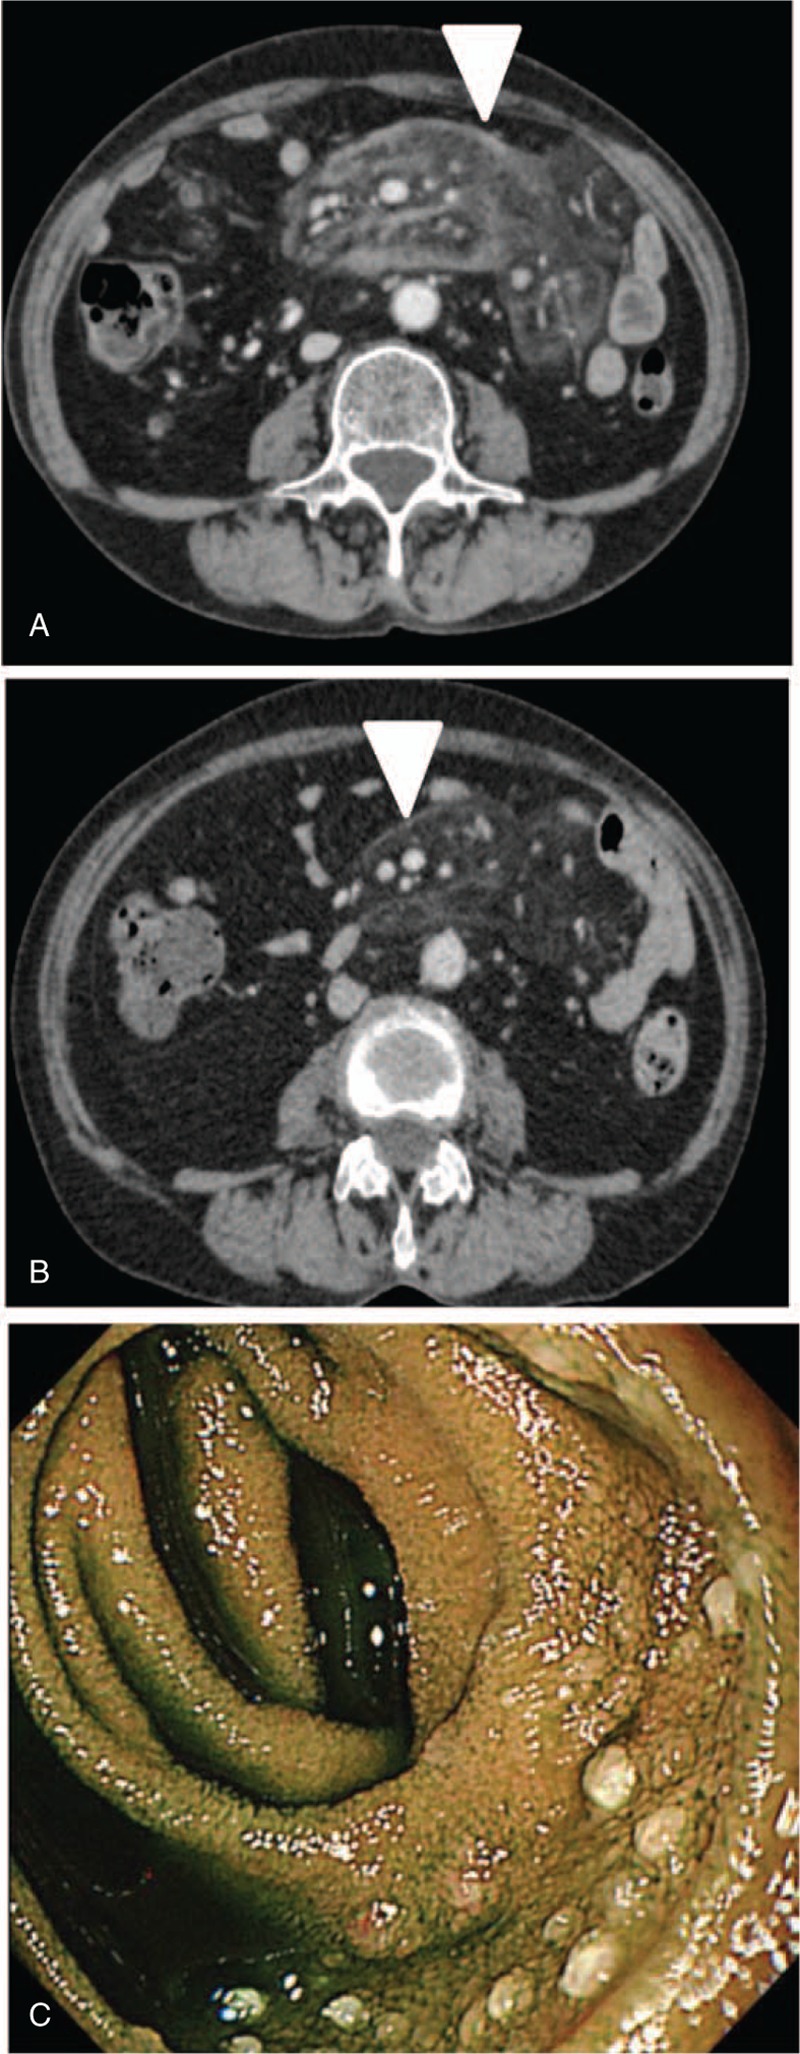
(A) Contrast-enhanced CT performed before steroid therapy showed increased density of mesentery (arrow head). (B) Single-balloon enteroscopy showed white villi in the jejunal mucosa. (C) Contrast-enhanced CT performed after steroid therapy showed improvement of contrast enhancement of mesentery (arrow head).
